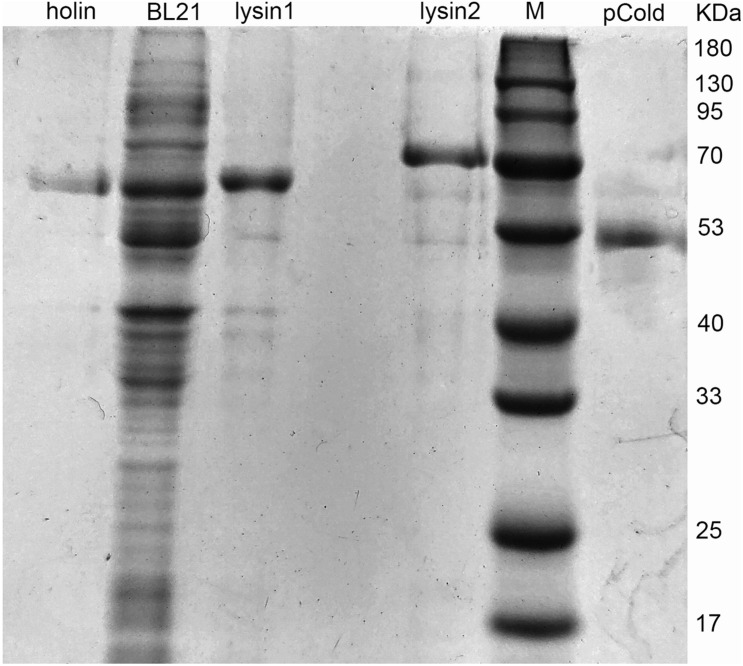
Protein expression of holin, lysin1, and lysin2. *E. coli* BL21 harboring the recombinant plasmids (pColdTF-holin, pColdTF-lysin1, pColdTF-lyisn2) were induced with 1 mM IPTG at 16°C for 16 h. After ultrasonic purification, the expressed proteins in the supernatant were collected and detected by SDS-PAGE.
